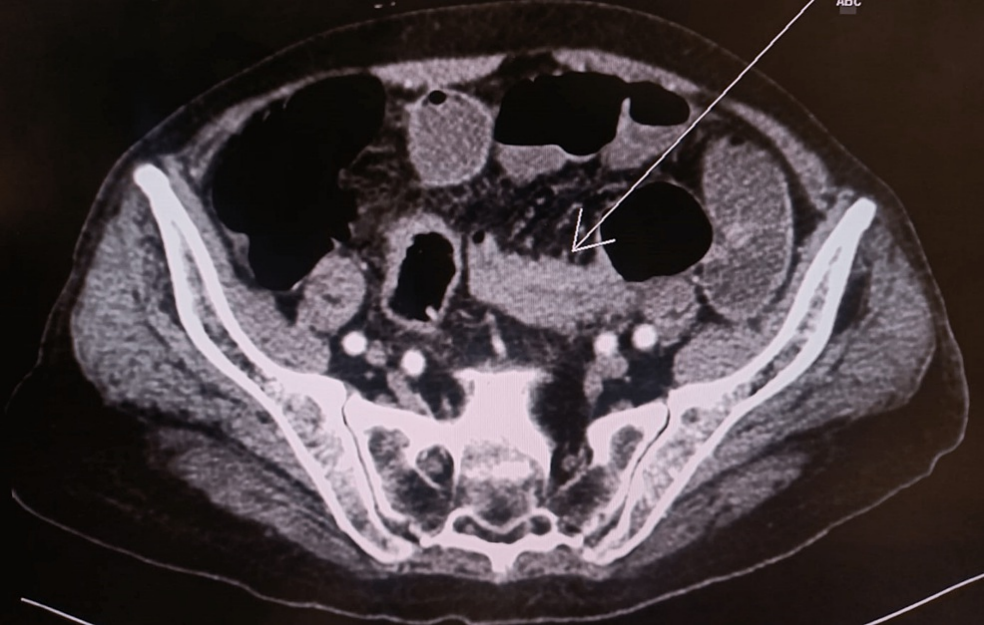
Abdominal CT scan showing a large obstructing solid mass arising from the sigmoid colon (arrow), measuring approximately 6 cm x 5.5 cm.
